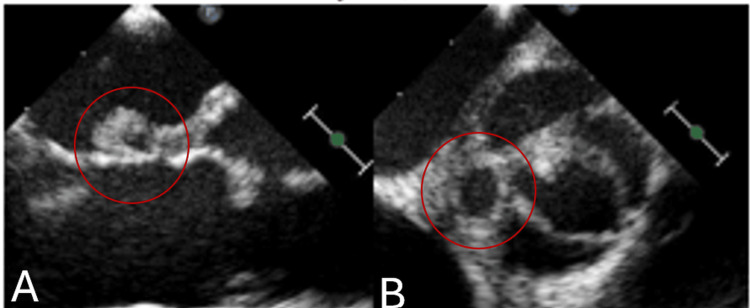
Transesophageal echocardiogram (TEE) revealing a mitral valve vegetation and an aortic sinus aneurysm with an associated abscess Parasternal long-axis and short-axis echocardiographic views, with red circles highlighting mitral valve vegetation (A) and aortic sinus aneurysm with an associated abscess (B).
